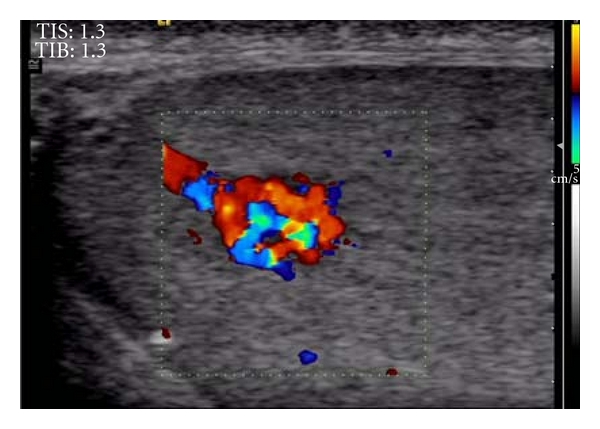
Color Doppler ultrasound demonstrates prominent vessels that entirely involve the mass.
